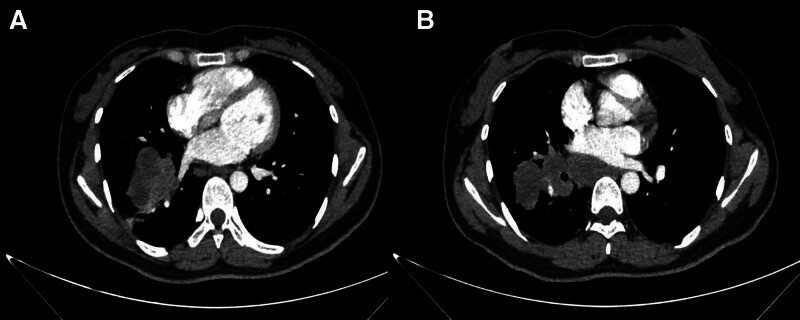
November 28, 2021. Lung computed tomography: masses in the right hilar and lower middle lobe of the right lung, considered malignant. Right hilar and mediastinal lymph node metastasis. (A) Primary tumor. (B) Maximum cross-section of tumor.
